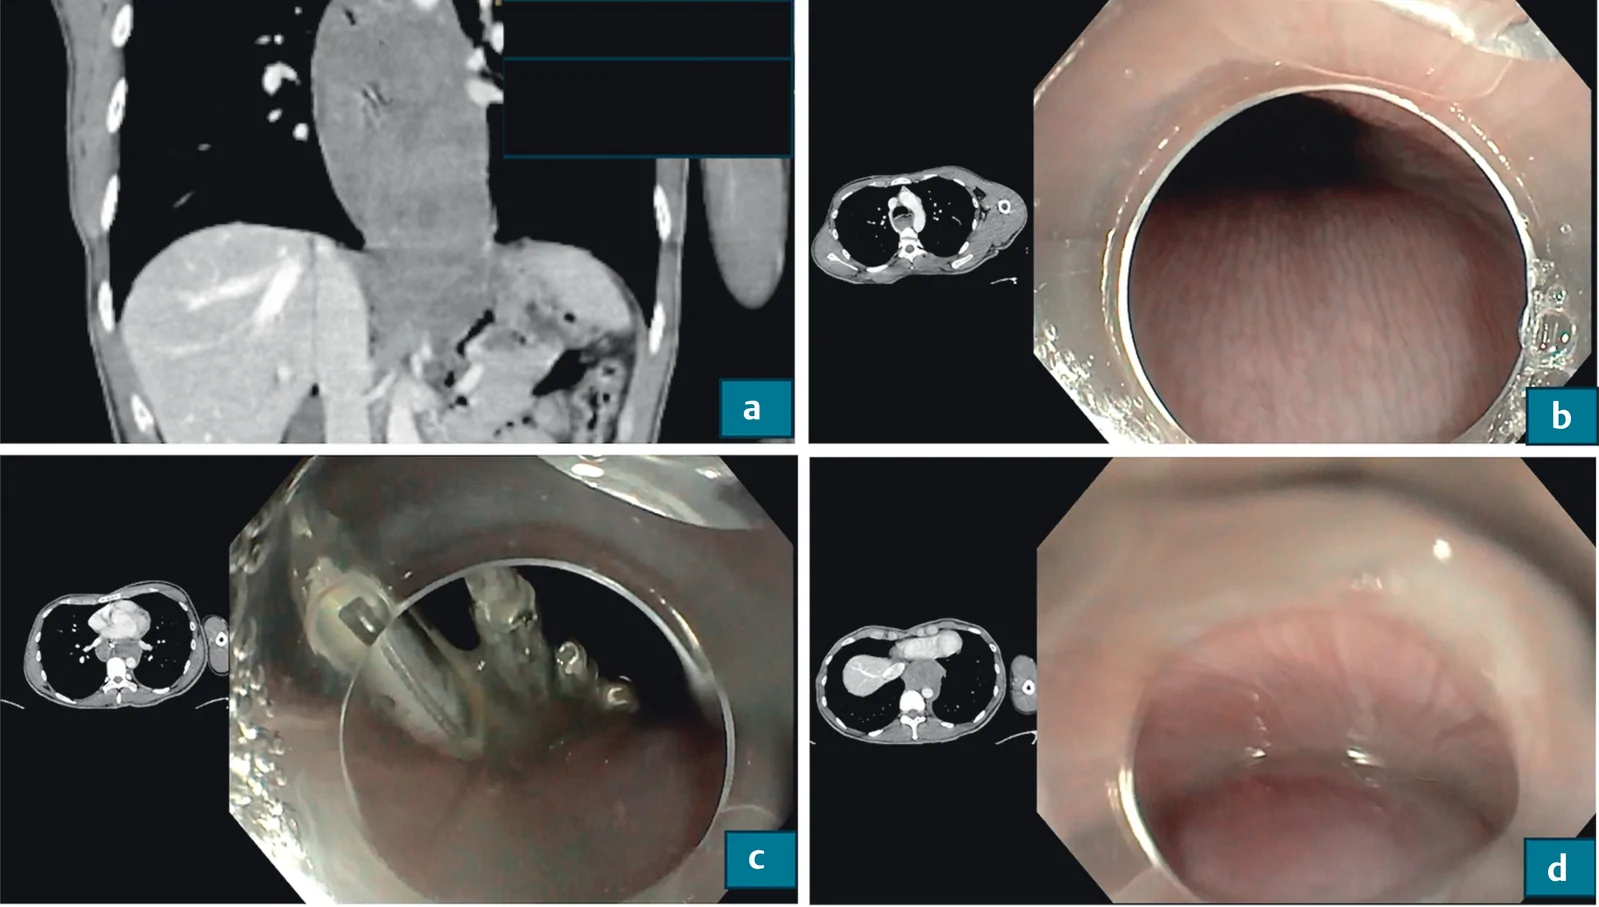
CT and endoscopic aspect of the hematoma: (
